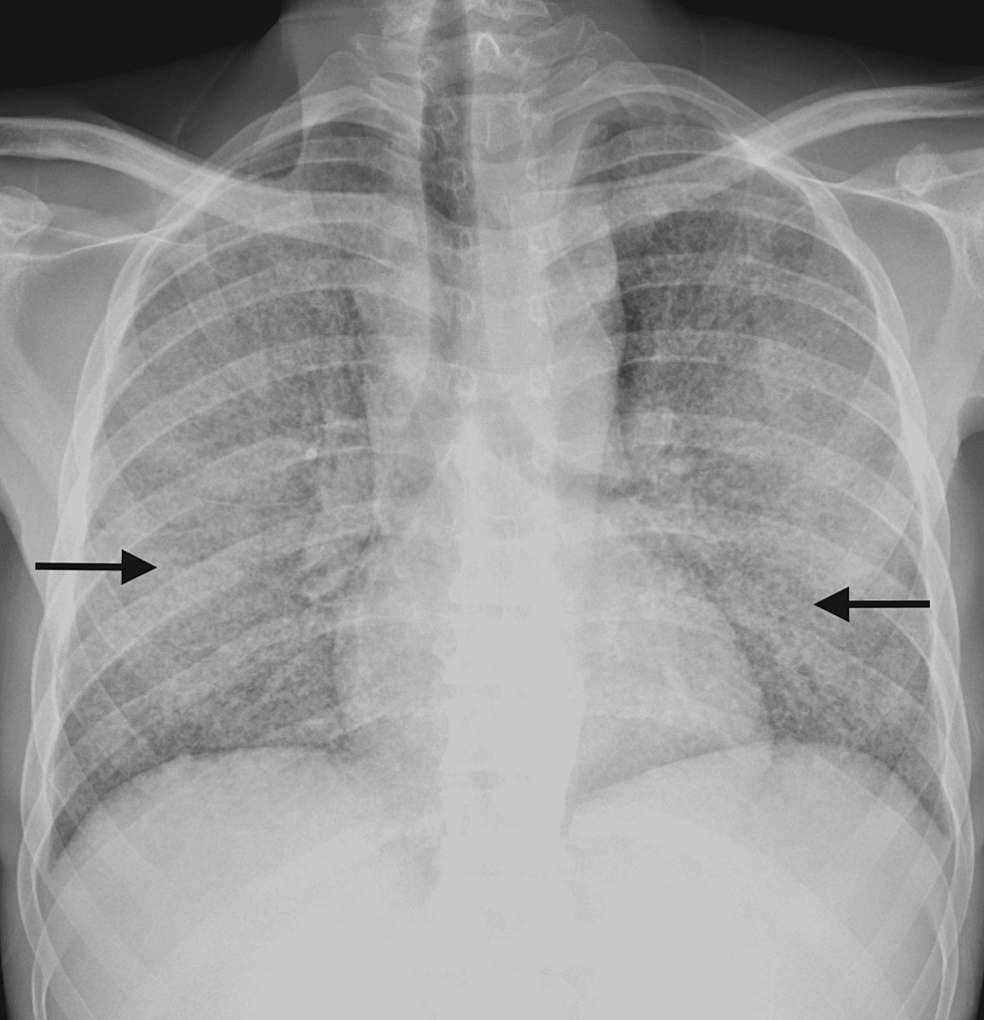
Thoracic X-ray on ICU admission The arrows show the interstitial alterations, in a bilateral diffuse distribution, which explained the clinical respiratory deterioration.
